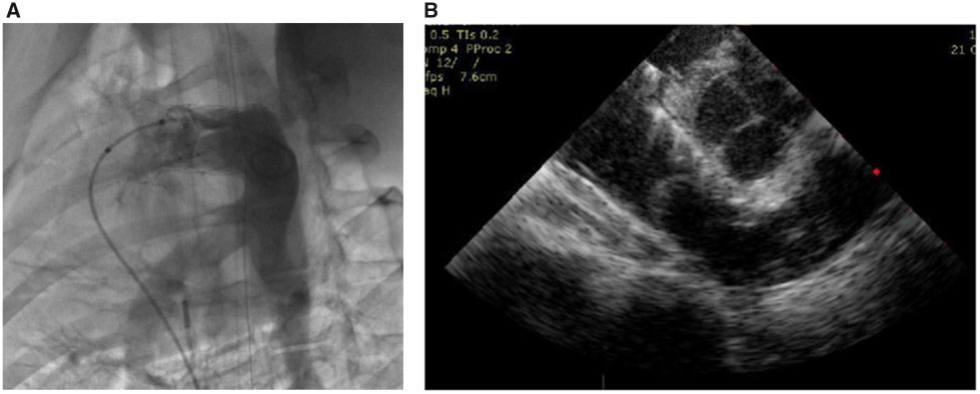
Angiography (A) and echocardiography (B) showed that the valve was positioned well and there was no obvious regurgitation.
